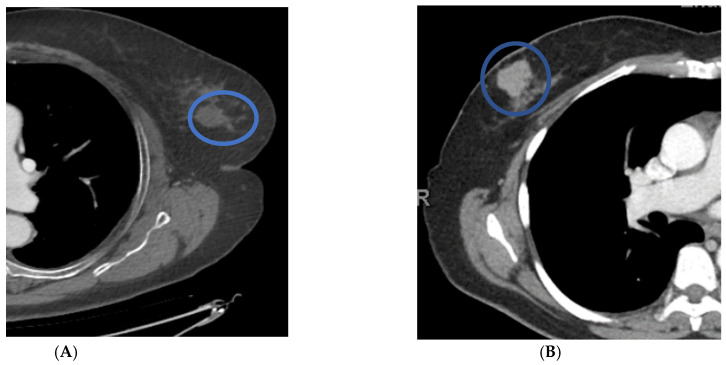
Distinguishing features of benign versus malignant masses on contrast-enhanced chest CT scans in axial projection. Patient (A) has a benign nodule with smooth borders and HUs of less than twenty. Patient (B) has a malignant nodule with lobular borders and HUs greater than twenty.
